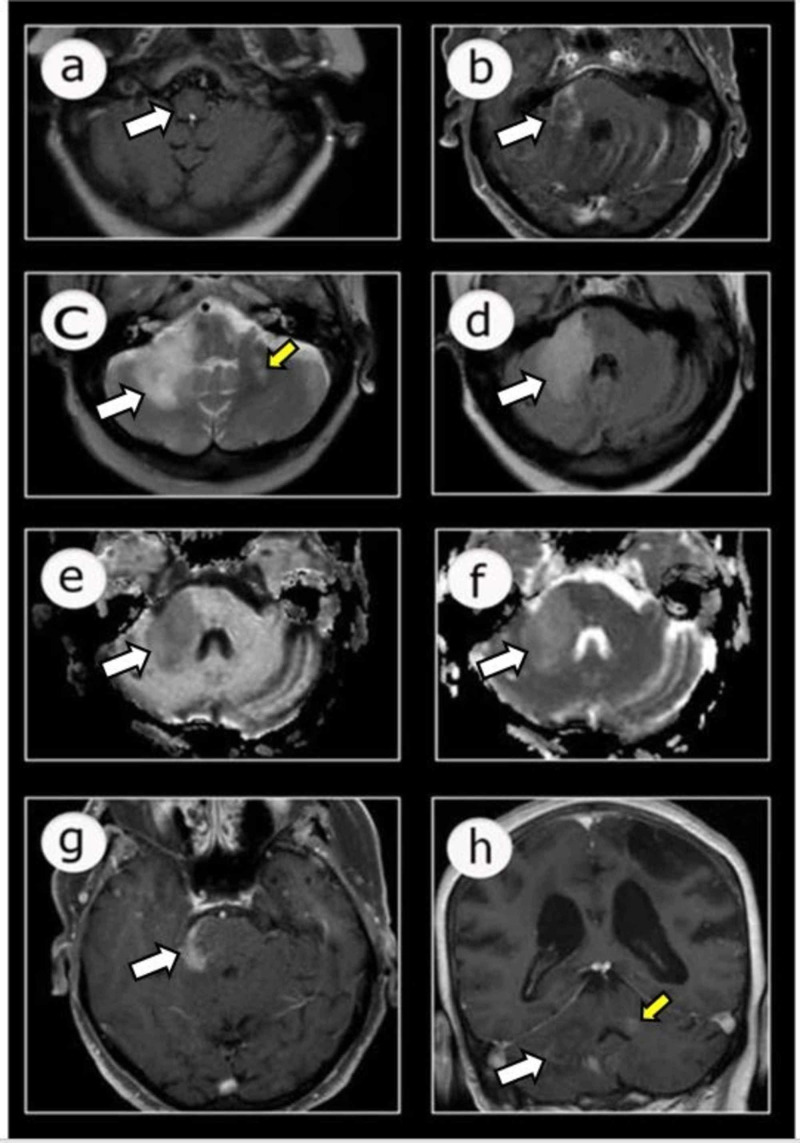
MRI of the brain with contrast showing an irregular right pontine isointense lesion on T1 (a), hyperintense signal on T2 (c), and FLAIR (d) sequences, with the extension of the lesion to the right cerebellar hemisphere showing minimal edema adjacent to the fourth ventricle. The lesion shows restriction on DWI (e) and ADC map (f) and heterogeneous enhancement at the margins (b, g, and h). Another T2 hyperintense smaller lesion is demonstrated at the left middle cerebellar peduncle and left cerebellar hemisphere (c) with heterogeneous enhancement (h). FLAIR, fluid-attenuated inversion recovery; DWI, diffusion-weighted images; ADC, apparent diffusion coefficient
